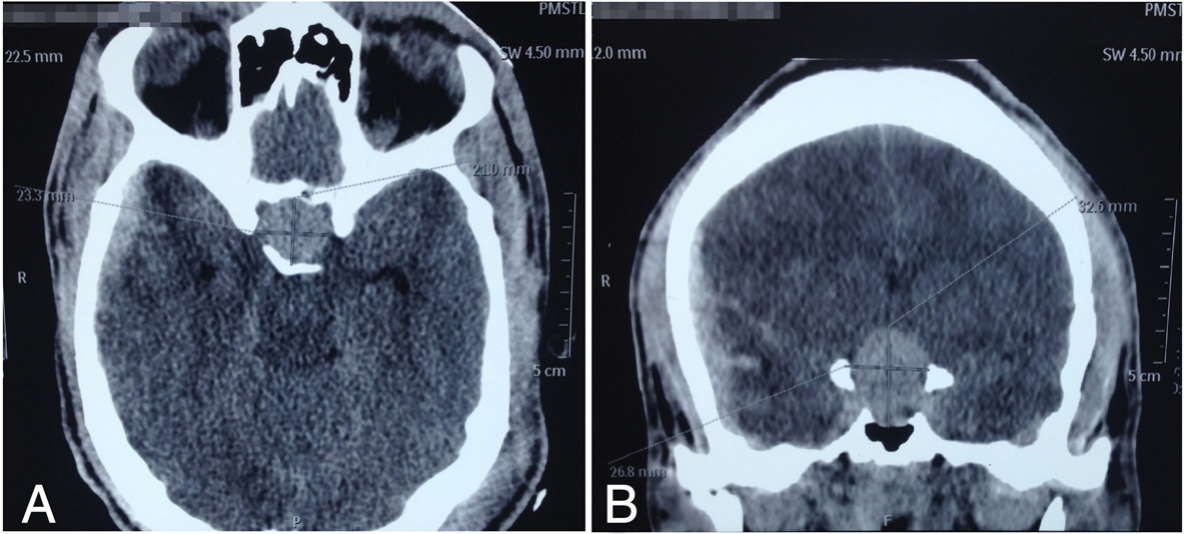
Initial computed tomography scan after head trauma. Axial (A) and coronal (B) computed tomography scans showing the patient’s pituitary macroadenoma, which was 21×26×32mm in size with suprasellar expansion.
